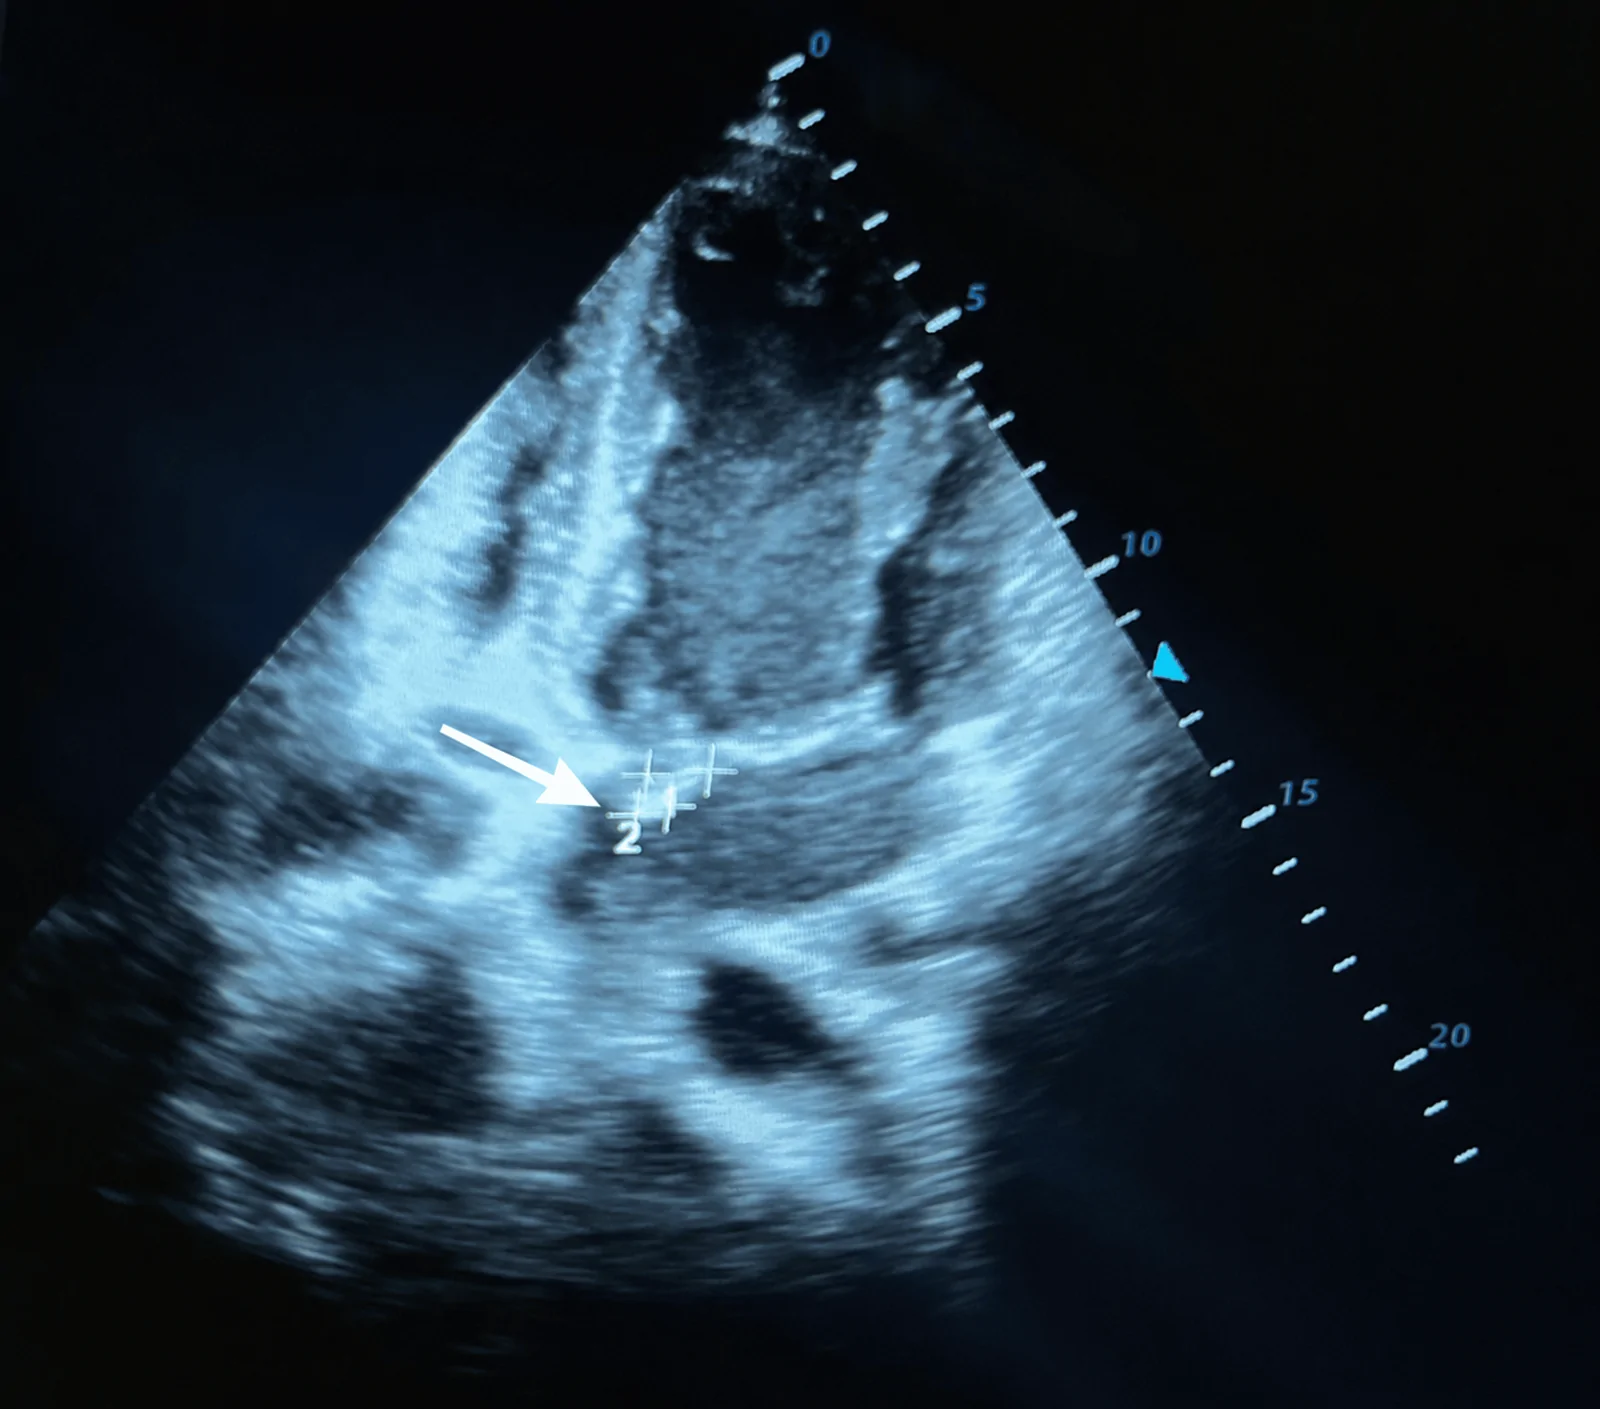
Transthoracic echocardiography showing aortic-valve vegetation in Case 2. A mobile aortic-valve vegetation measuring 13.2 × 6.5 mm is shown. The white arrow indicates the vegetation.
